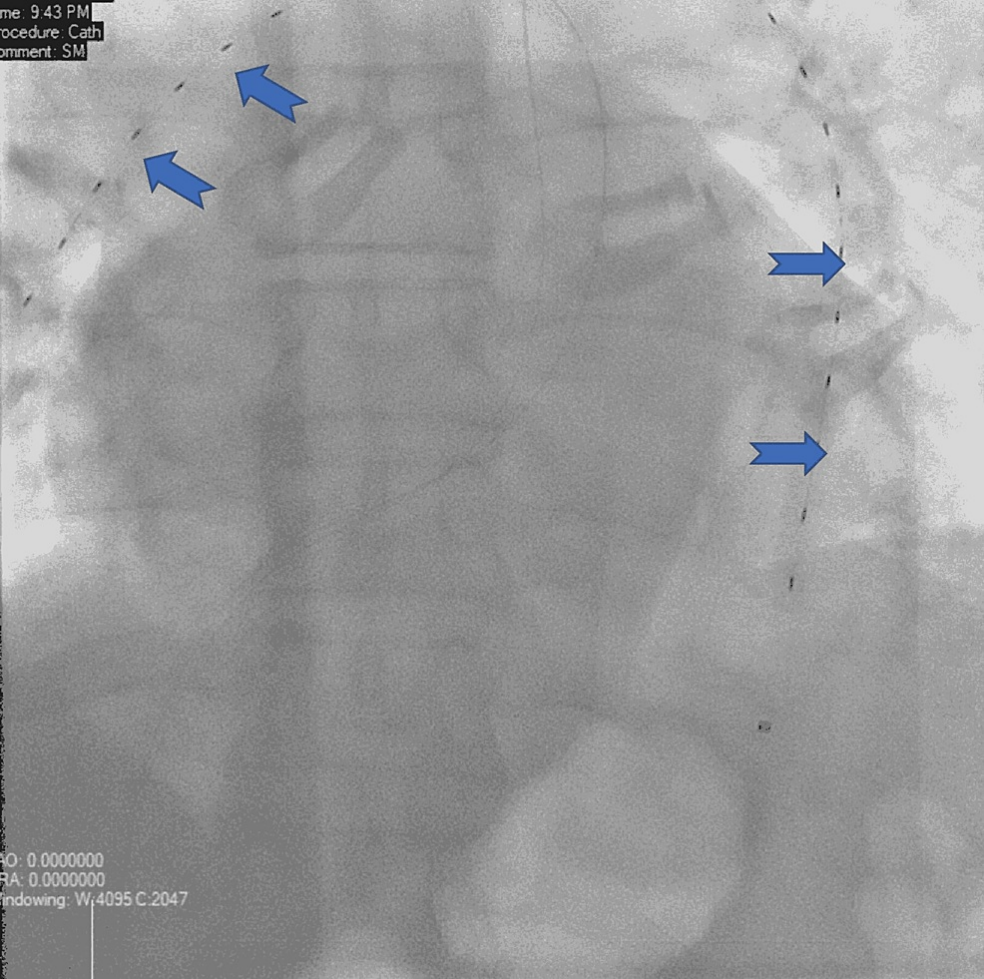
EkoSonic™ endovascular system-directed thrombolysis catheter system with ultrasound transducers used to direct thrombolytic agents at pulmonary embolism.
